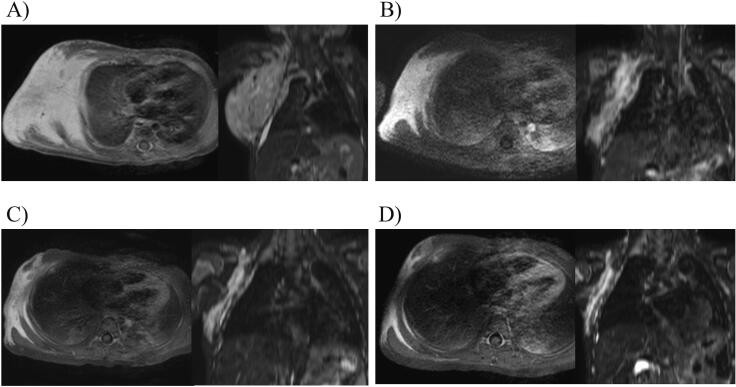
Magnetic resonance imaging of the lesion during treatment. Axial and coronal T2-weighted magnetic resonance imaging of the right chest demonstrates the huge mass infiltrating the right pleura and lung. Before treatment, the tumor compressed the right chest. After treatment, compression from the tumor had reduced. (A) Prior to treatment, (B) 3 months after treatment, (C) 6 months after treatment, and (D) 12 months after treatment.
